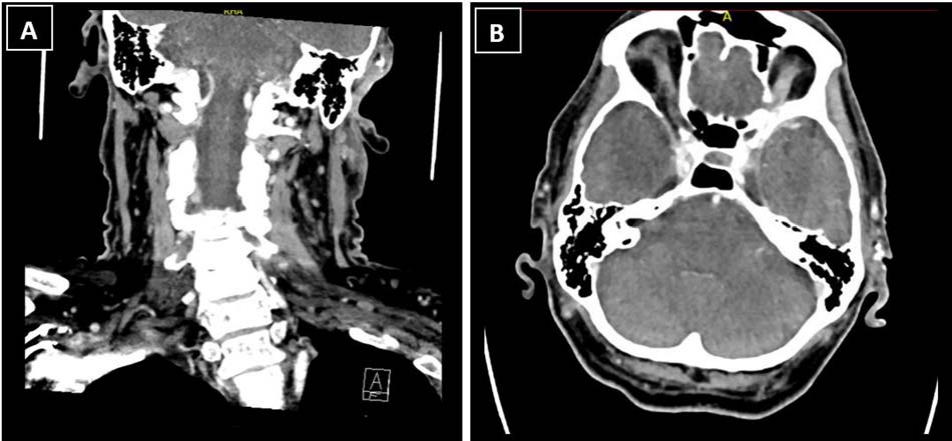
CT neck showed a 1.3 × 1.2 × 1.3-cm ill-defined soft tissue enhancement above the left external auditory canal involving the subcutaneous tissue and overlying skin; (A) coronal view; (B) axial view.
